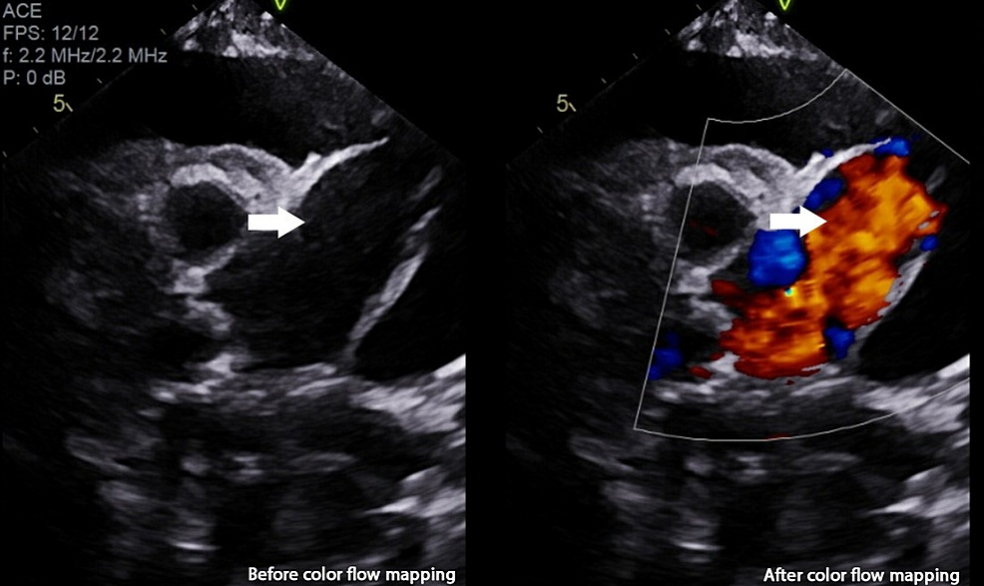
Aortic root on TTE seen severely dilated with dissection flap separating it into true and false lumen. On color Doppler imaging, blood is seen circulating within the true lumen. TTE: transthoracic echocardiography
